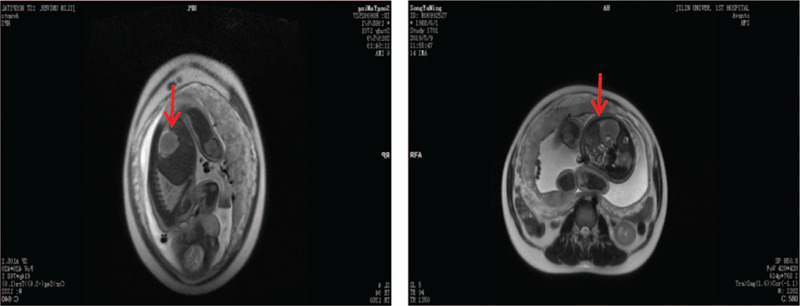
MRI shows that the posterolateral contour of the right kidney of the fetus is plump, locally convex, and the signals of TIWI, T2-haste and T2-trufi are similar to those of the renal parenchyma. The signals of DWI are slightly higher than those of the renal parenchyma, and the boundary between the lesion and the adjacent structures is clear. DWI = diffusion weighted imaging.
